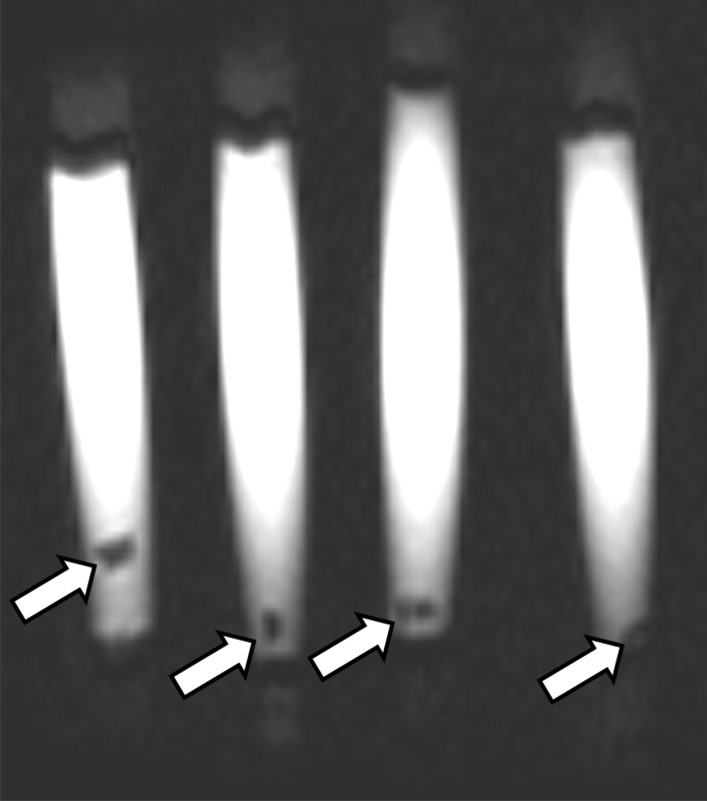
In vitro MRI of xanthine urinary stones: coronal T2-weighted MR image shows stones (arrows) as hypointense signal voids within hyperintense saline-filled syringes
